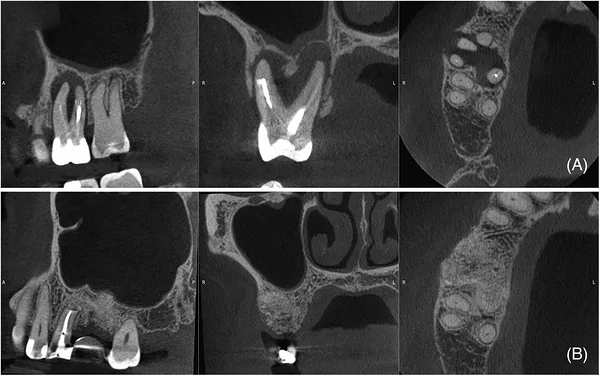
Case 2: Initial CBCT (sagittal, coronal, axial) showing hypodense lesion around teeth #3 and #4, buccal cortical bone fenestration and thickening of the sinus membrane (A). CBCT at 10 months postoperatively (sagittal, coronal, axial), showing bone formation, apical remodeling of tooth #4, preserved tissue volume and normalized sinus membrane (B). CBCT, cone‐beam computed tomography.
